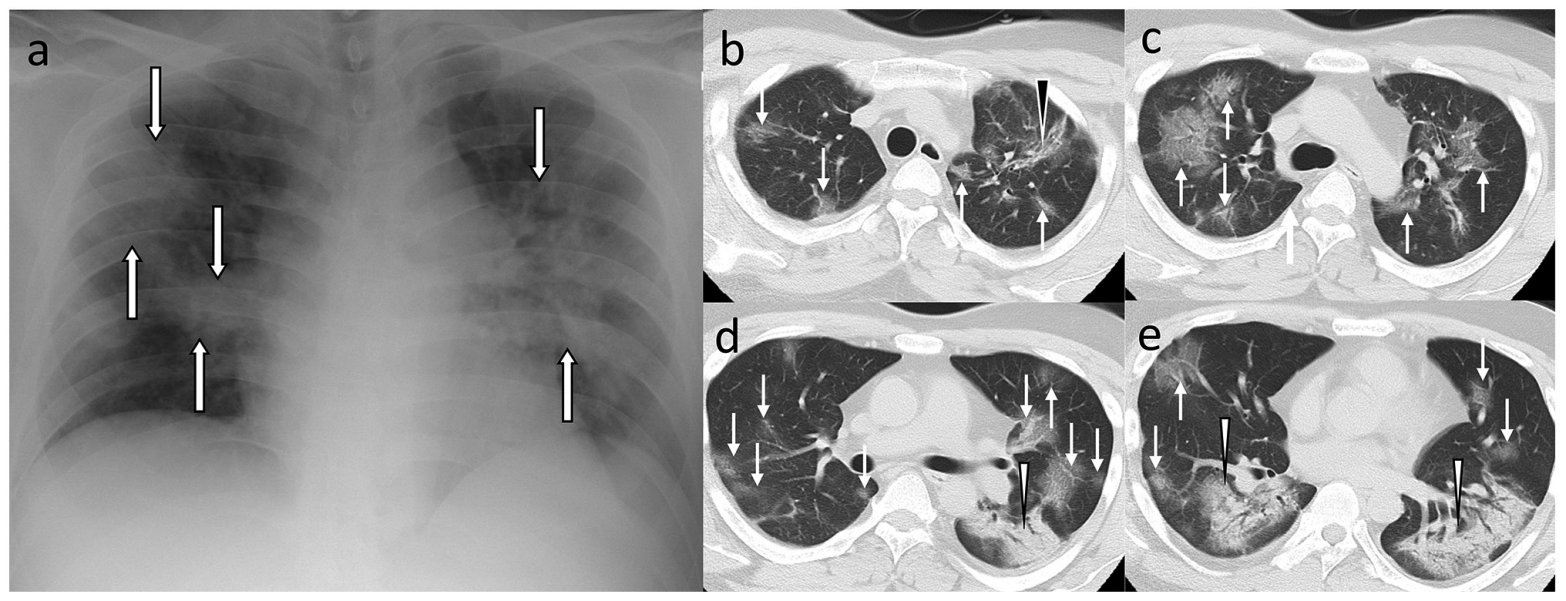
Exacerbation of COVID-19 pneumonia in a 30-year-old man. (a) Chest radiography revealed patchy infiltrations in the bilateral lung (indicated using arrows). (b-e) CT image demonstrated numerous ground-glass opacities (indicated using white arrows) in the whole lung, linear opacity associated with ground-glass opacity [(b) black arrowhead], and consolidation with air bronchogram in the bilateral lower lung lobe [(d and e) white arrowheads]. CT, computed tomography.
